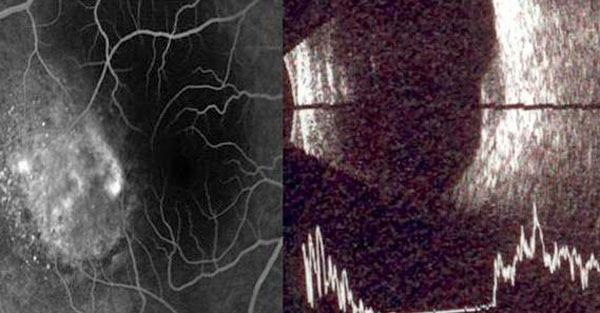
(a) Fundus fluorescein angiography of the right eye. (b) B-scan Ultra sonogram of the posterior segment.
